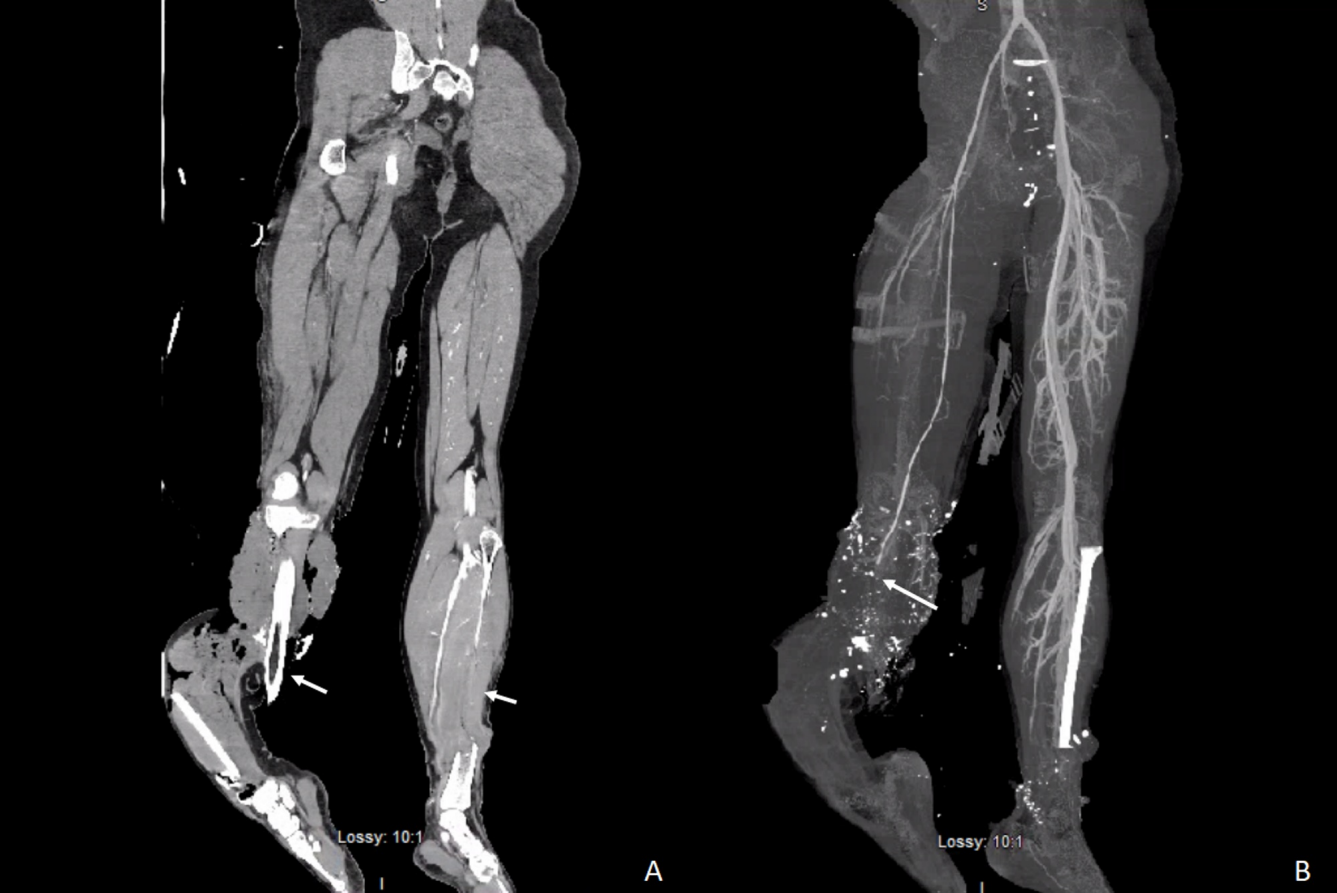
(A) CT of lower extremities without contrast showing bilateral compound fractures of tibia and fibula (white arrows). Right lower extremity was later amputated. (B) Vascular study showing interruption of tibial artery in right lower extremity (white arrow).
